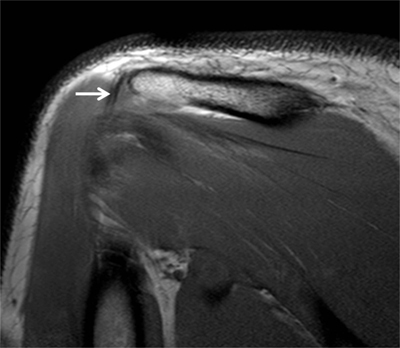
Subacromial pseudospur. Coronal oblique PD-weighted MR image depicts the normal attachment of the tendon of the deltoid muscle visible on one single section mimicking an enthesophyte (arrow). Analysis of consecutive coronal oblique MR images is necessary to avoid misinterpretation.
